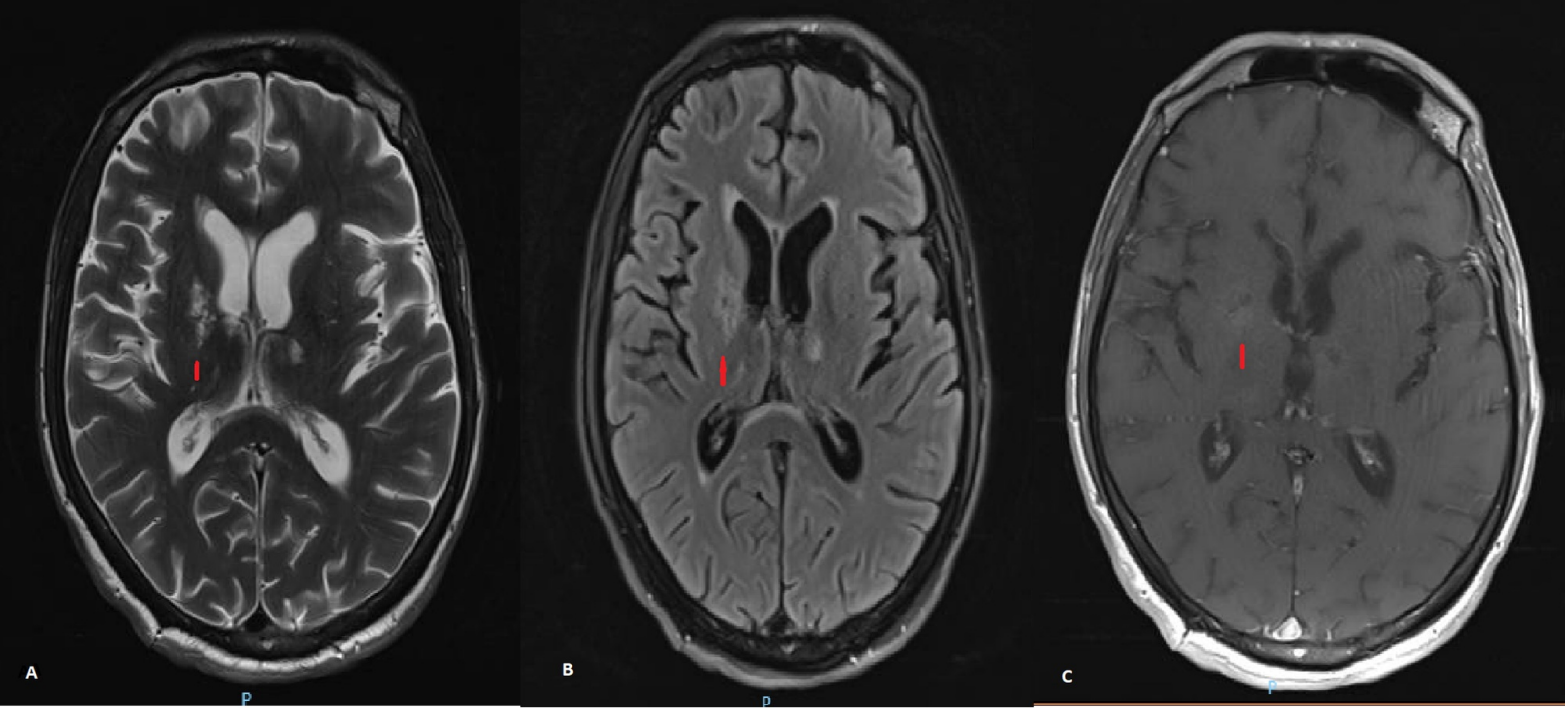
Brain magnetic resonance imaging (MRI) six weeks after mirtazapine therapy A) T2-weighted images (T2WI) showing marked resolution of previously seen lesion over gray matter; B) fluid-attenuated inversion recovery (FLAIR) image demonstrating similar resolution of lesion size; C) contrast study shows no discernible area of contrast uptake.
